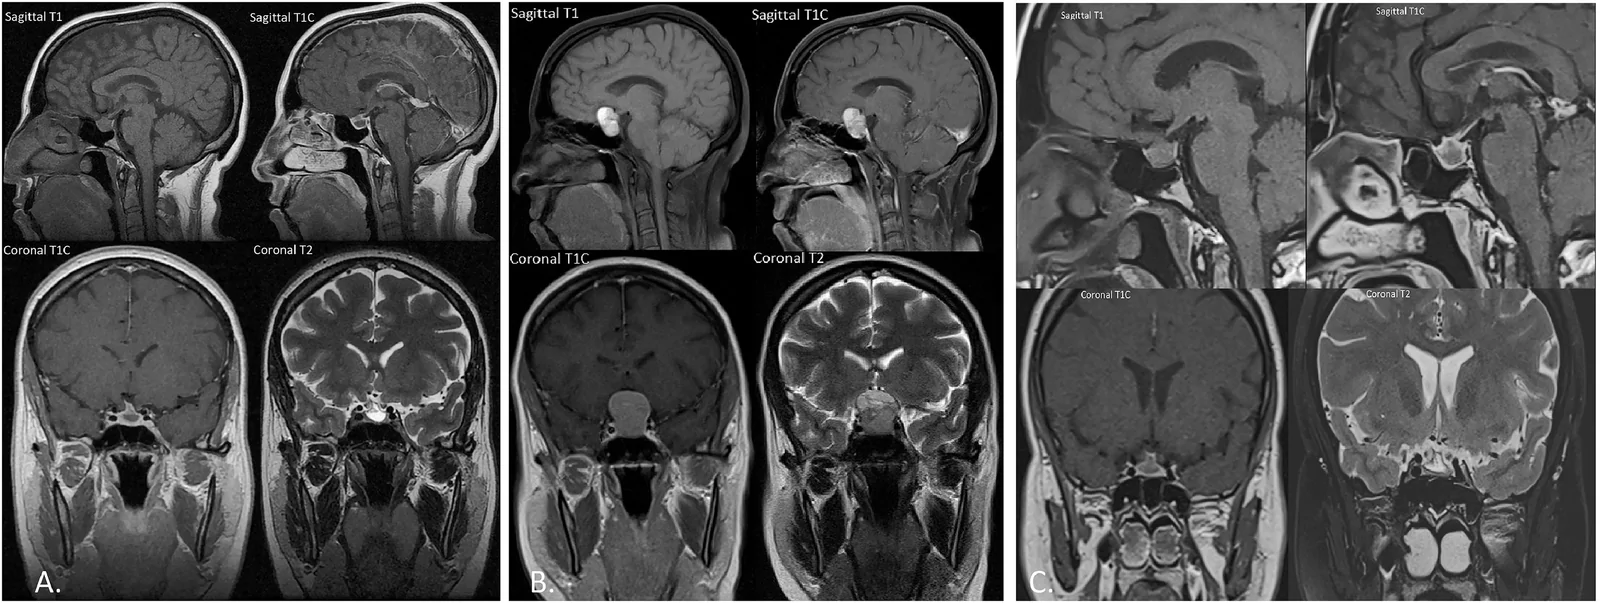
(A) The patient's pituitary MRI before her third pregnancy. (B) Massive enlargement of the tumor compared to section A is seen. (C) Pituitary MRI of the patient after the delivery, compared to section B, shows a regression of tumor size into a microadenoma. T1C; T1-weighted MRI with contrast.
